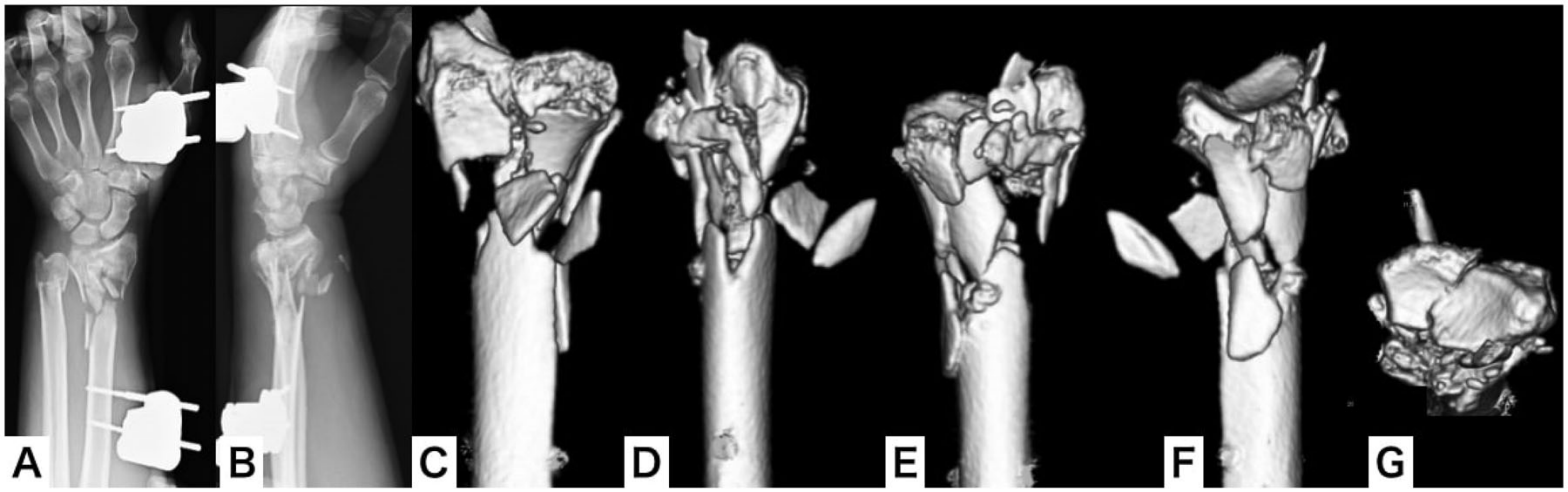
Plain x-ray and three-dimensional computed tomography (3-D CT) of the left wrist after external fixation as a first-line treatment. (A,B) Plain x-ray of the left wrist; (C–G) 3-D CT of the left wrist.
